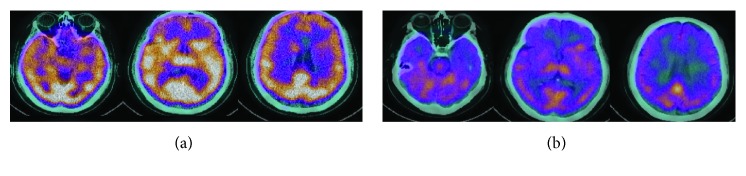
Colour scale brain perfusion SPECT analysis with 99mTx-HMPAO fused with CT scan for patient 2: (a) April 2018; (b) August 2018.
